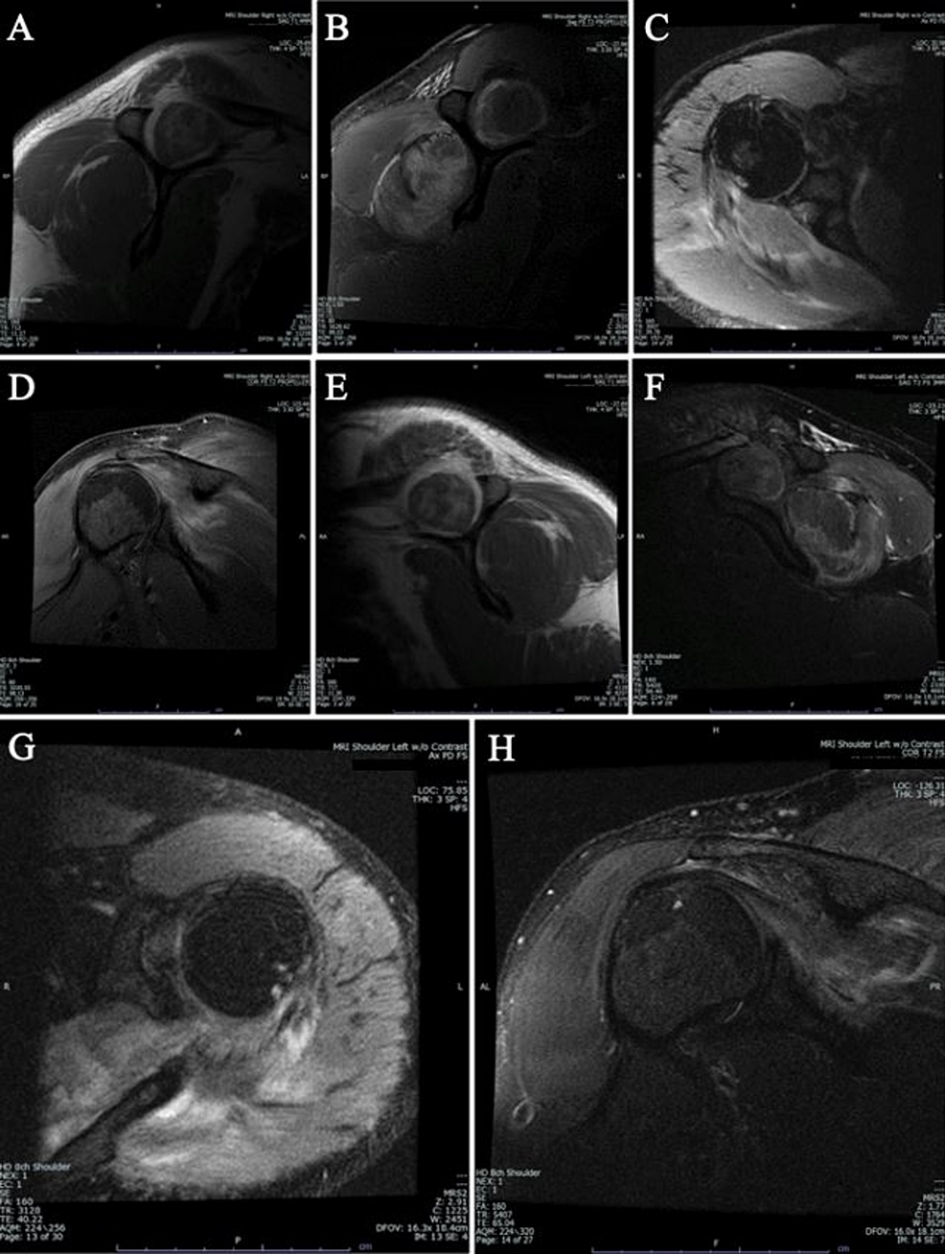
Postoperative bilateral shoulder rhabdomyolysis following cardiothoracic surgery confirmed with MRIs of bilateral shoulders without contrast. (A) Sagittal oblique T1-weighted MRI of the right shoulder. (B) Sagittal oblique T2-weighted MRI of the right shoulder. (C) Axial MRI of the right shoulder. (D) Coronal oblique T2-weighted MRI of the right shoulder. (E) Sagittal oblique T1-weighted MRI of the left shoulder. (F) Sagittal oblique T2-weighted MRI of the left shoulder. (G) Axial MRI of the left shoulder. (H) Coronal oblique T2-weighted MRI of the left shoulder. MRIs in multiple planes of bilateral shoulders demonstrate intramuscular hemorrhage involving the supraspinatus muscles bilaterally, prominent intramuscular edema of the infraspinatus, and teres minor muscles bilaterally, and mild edema in the posterior deltoid and trapezius muscles bilaterally.
